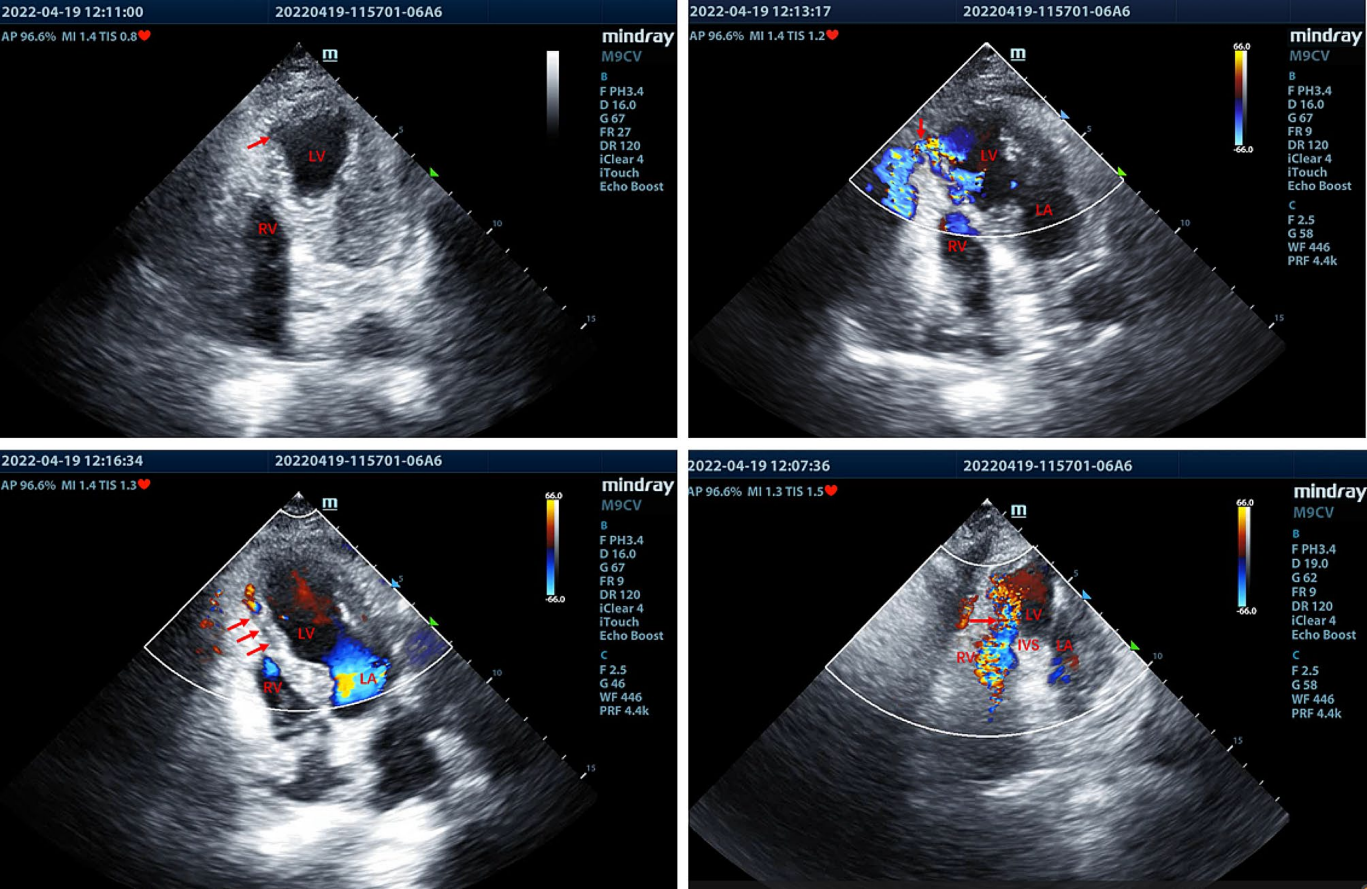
Bedside transthoracic echocardiography revealed ventricular aneurysm formation at the apex of the left ventricle (A) (red arrow), ventricular apical wall rupture (B) (red arrow) and ventricular septal myocardial dissection (C) (red arrow) and interventricular septum (IVS) rupture (D) (red arrow)
